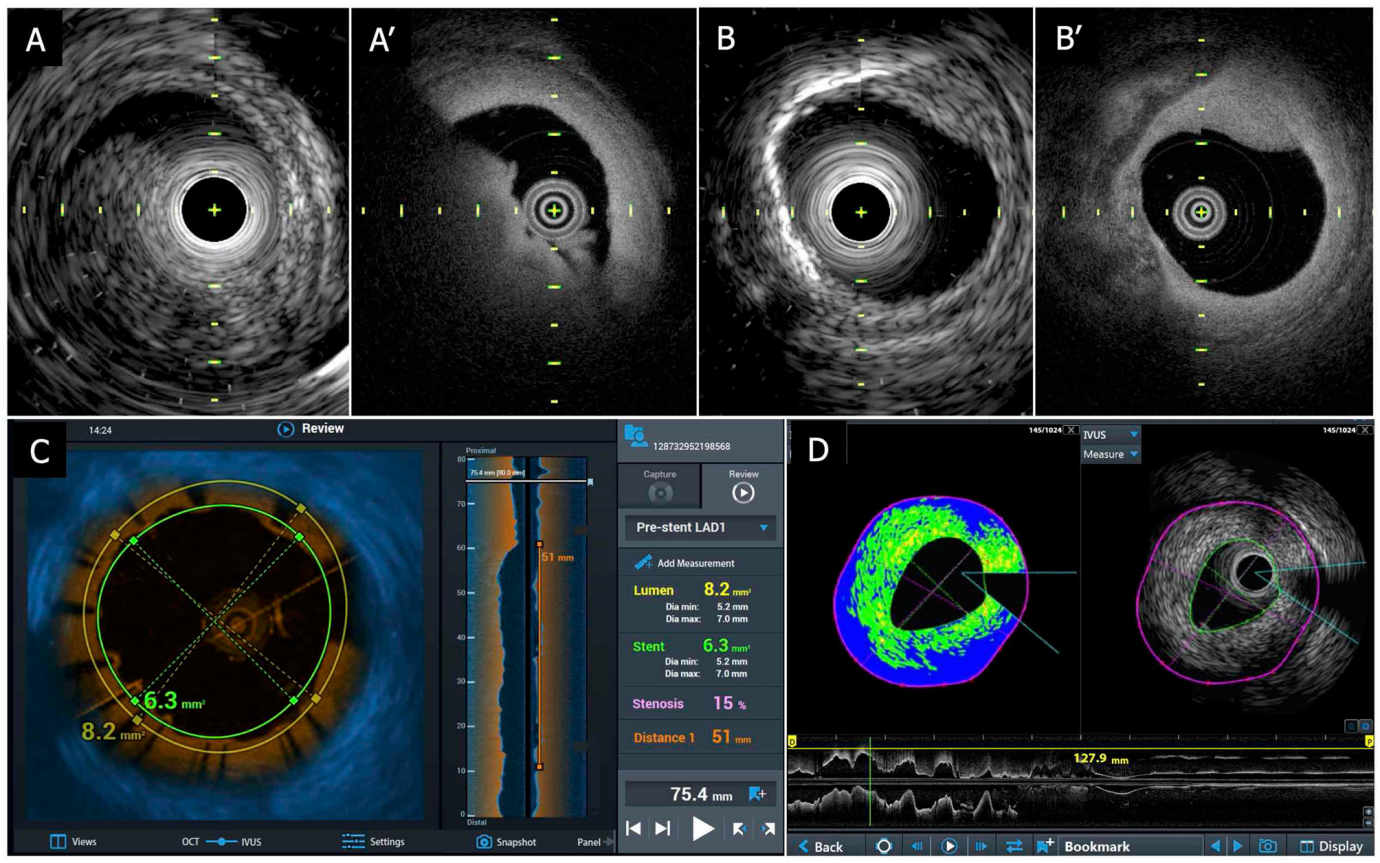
TERUMO hybrid IVUS–OCT catheter system; sample images. (A) Coregistered intracoronary imaging of thrombus in IVUS (A-1) and OCT (A-2) in a cadaver coronary artery. (B) Coregistered intracoronary imaging of calcification in IVUS (B-1) and OCT (B-2) in a cadaver coronary artery. (C) Fusion image of IVUS and OCT. (D) IB-IVUS image with OCT. IVUS, intravascular ultrasound; OCT, optical coherence tomography; IB, integrated backscatter.
